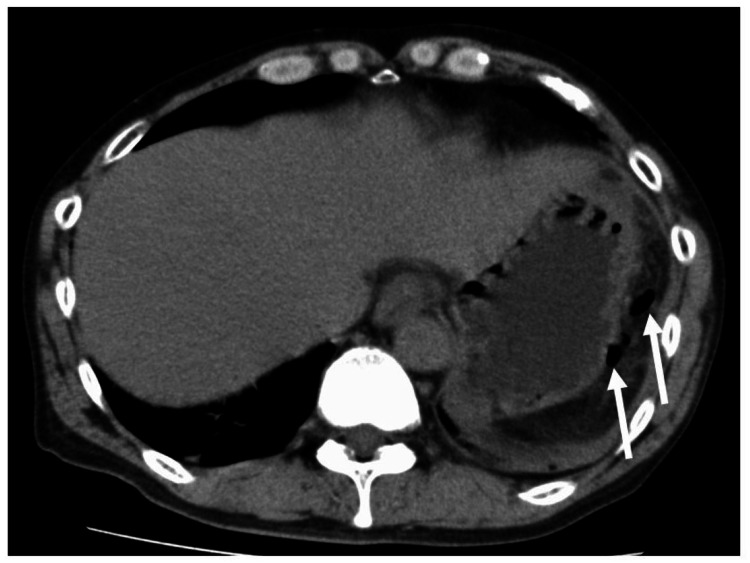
Computed tomography two months after double-pigtail plastic stent placement. Two months after double-pigtail plastic stent placement, fever and abdominal pain developed. Computed tomography revealed abdominal free air (arrow) and increased fat tissue density around the fistula formation site.
